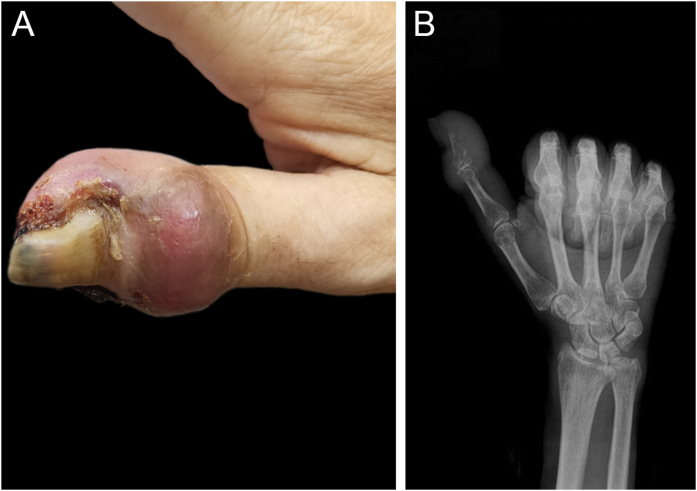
(A) In the distal phalanx of the right thumb, there was a purplish-red swelling encircling the finger, accompanied by erosions of the nail folds, thickening of the nail plate, discoloration, and morphological irregularities. (B) Radiographic imaging revealed extensive bone destruction and a loss of the anatomical integrity of the distal phalanx.
